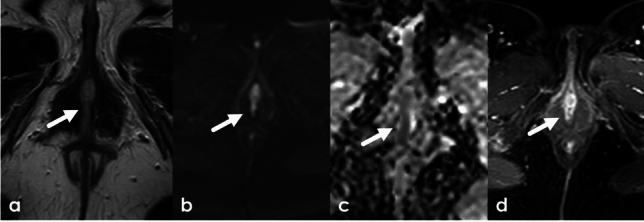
64-Year-old female with visible ulcerated erosive vulvar lesion. Axial T2WI demonstrates a T2 intermediate midline vulvar mass (a) with diffusion restriction (b, c) on DWI/ADC, and enhancement on axial and post-contrast images (d). Biopsy confirmed a diagnosis of squamous cell carcinoma
